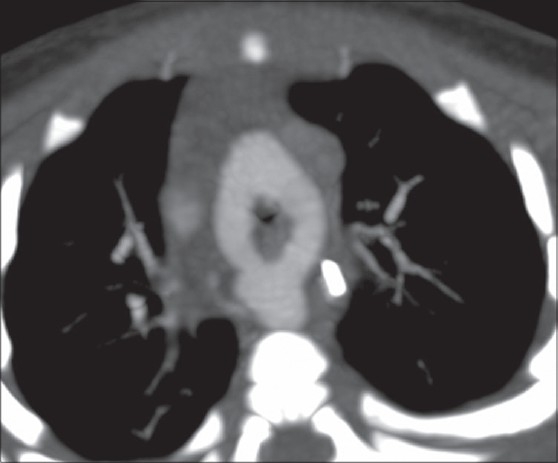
Axial CT chest shows double aortic arch
